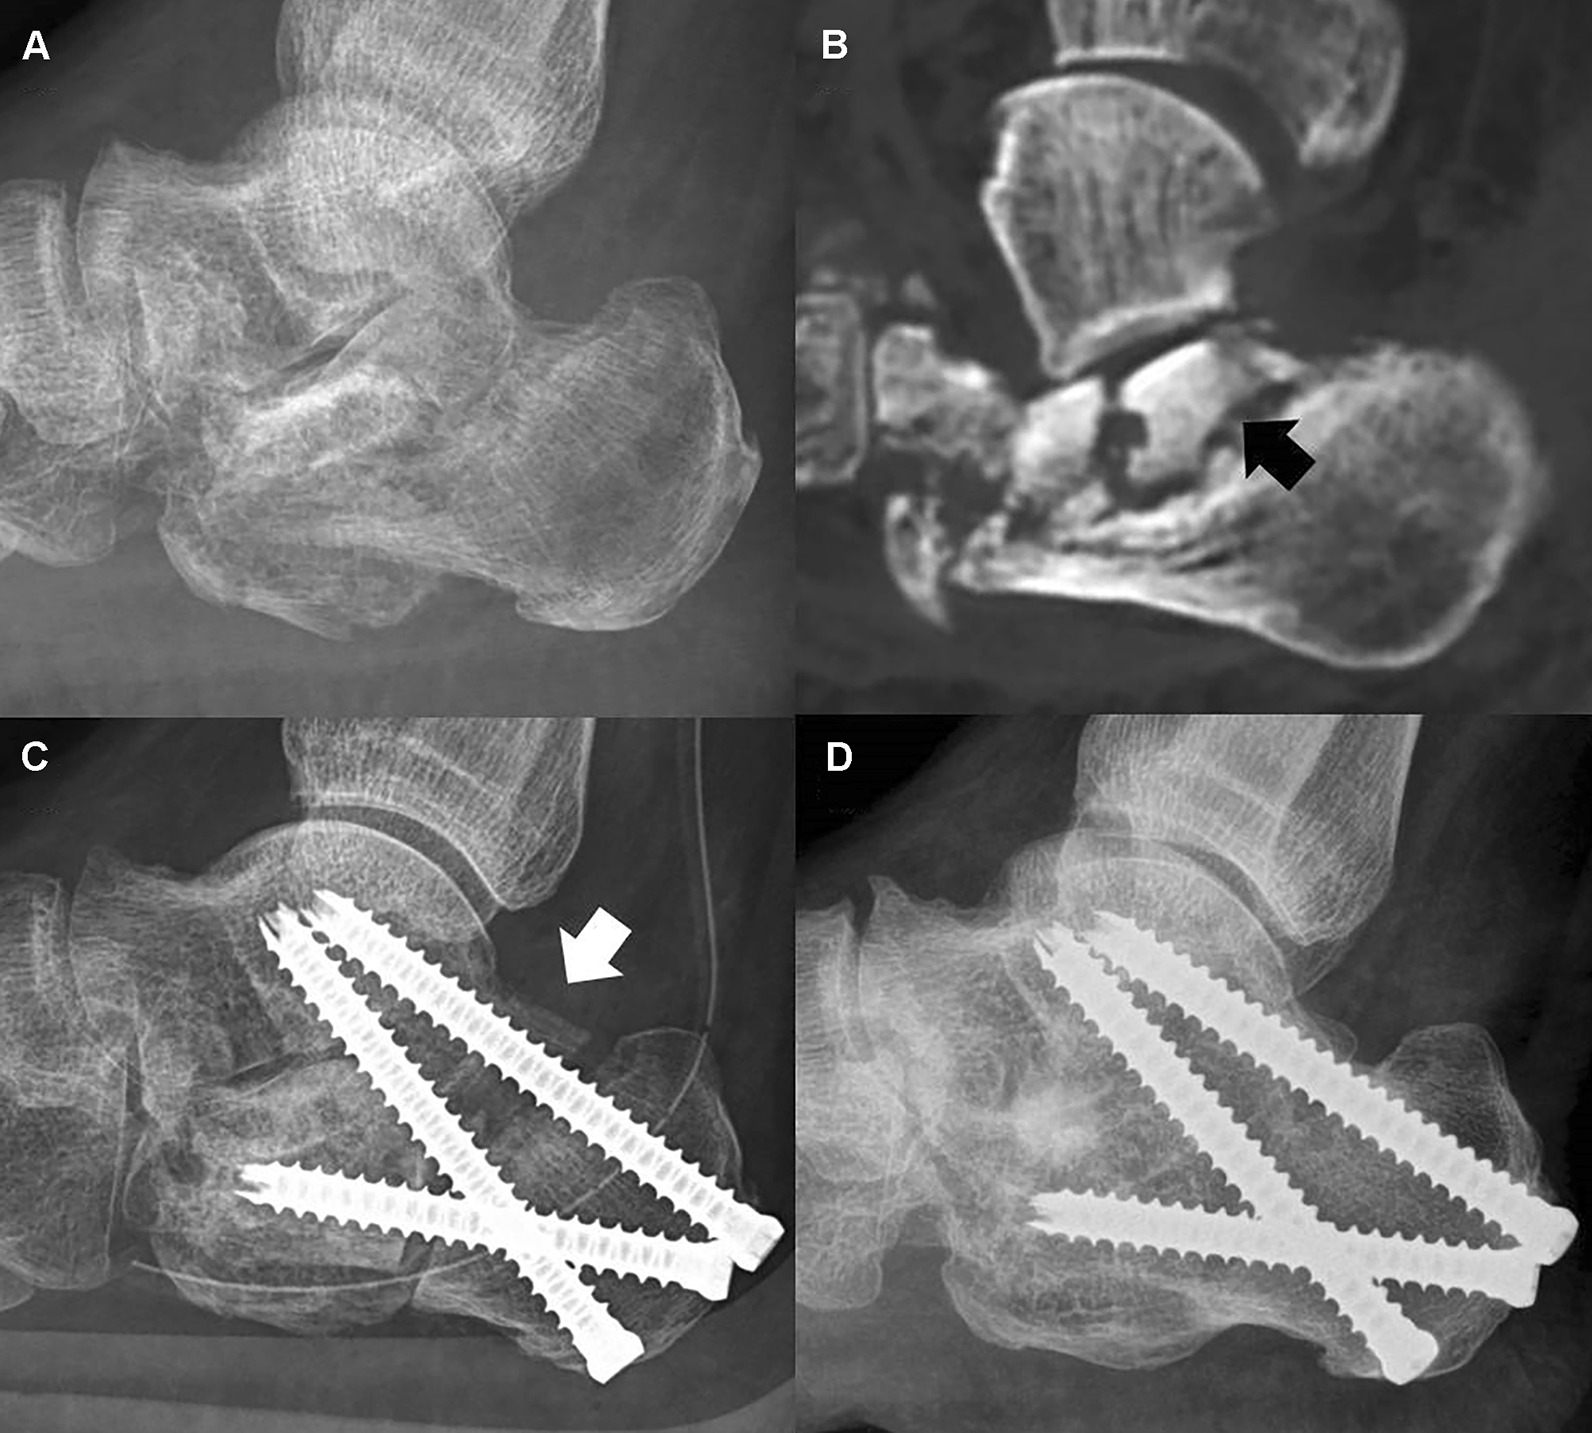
Avascular bone (black arrow) at the posterior facet of the calcaneus was observed on the a plain radiograph and b CT scan image after the pin fixation of a calcaneal fracture. c A large talocalcaneal gap left behind after removal of the avascular bone was filled with autoiliac tricortical bone (white arrow) which was fixed with two fully threaded screws. d Osseous consolidation was confirmed on the plain radiograph
